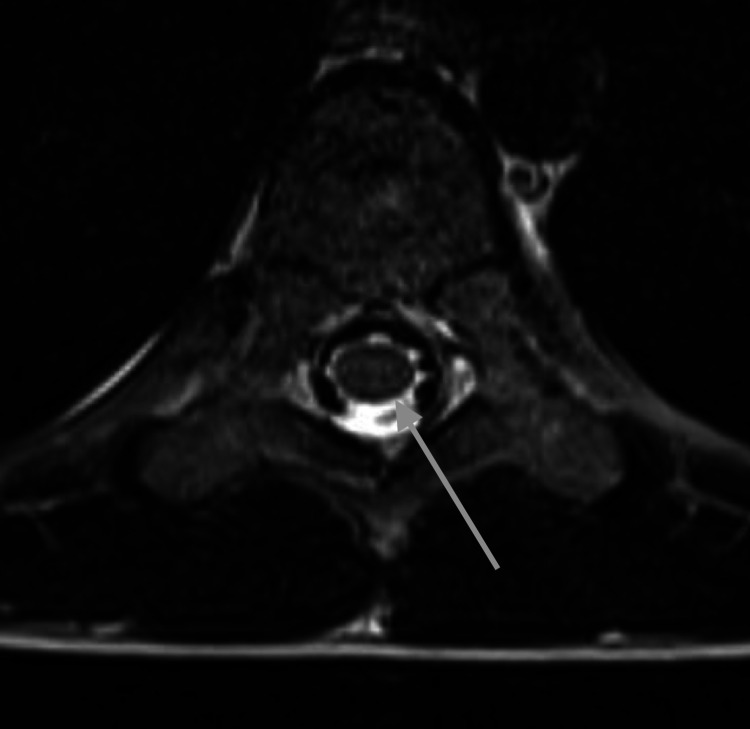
Representative axial T2-weighted image of the spine at the level of T9 at eight years of age shows resolution of the slit-like syrinx (gray arrow)
